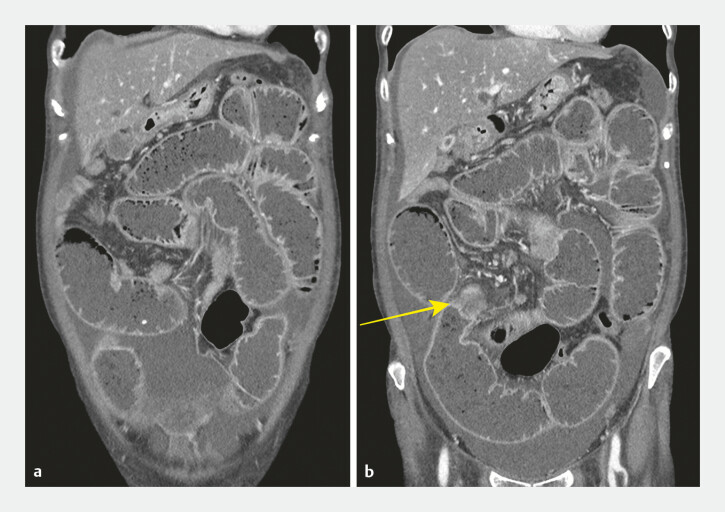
Initial computed tomography images showing:
